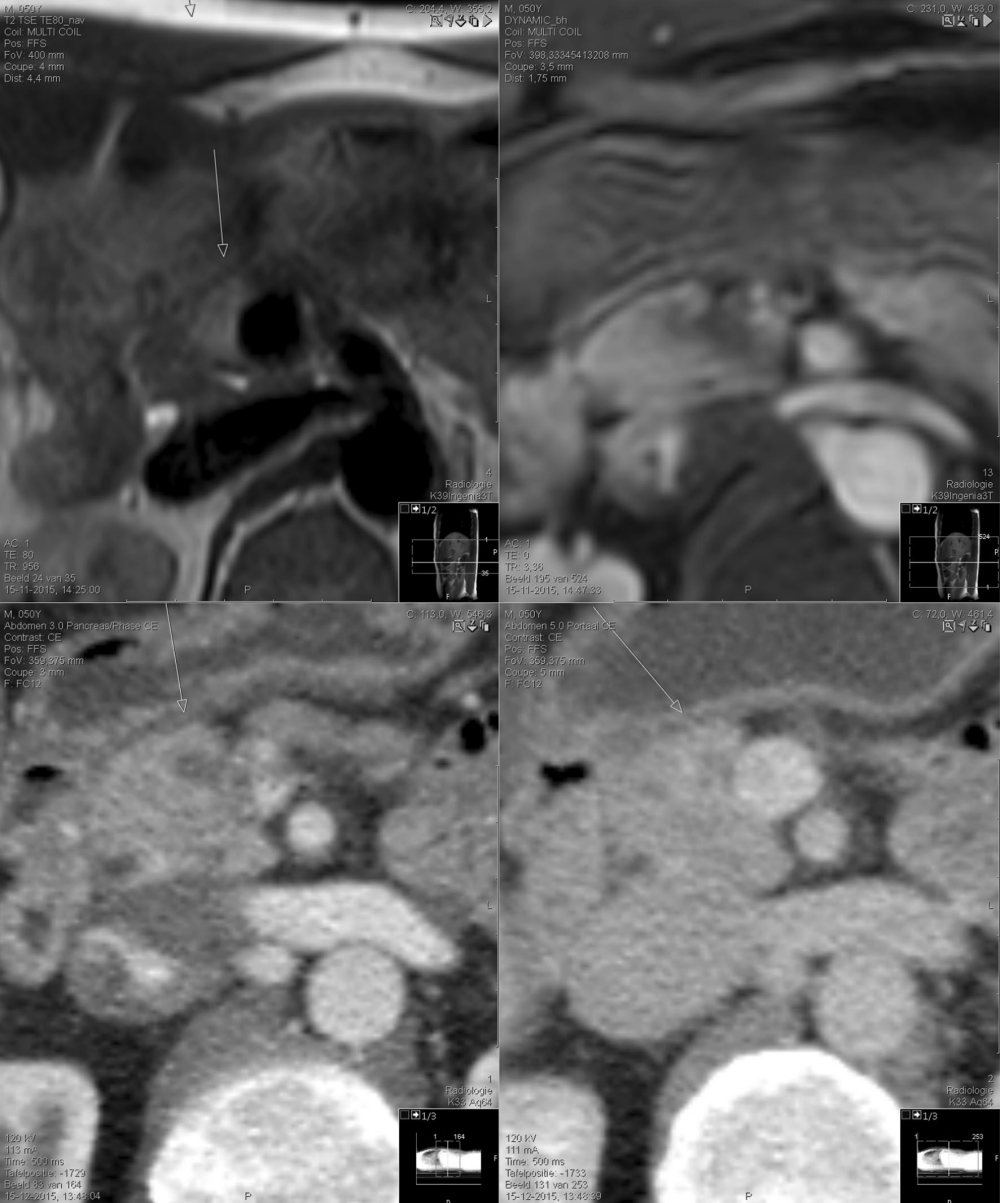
MRI (*upper panel*) and CT-scan (*lower panel*) of the pancreas in case 2
